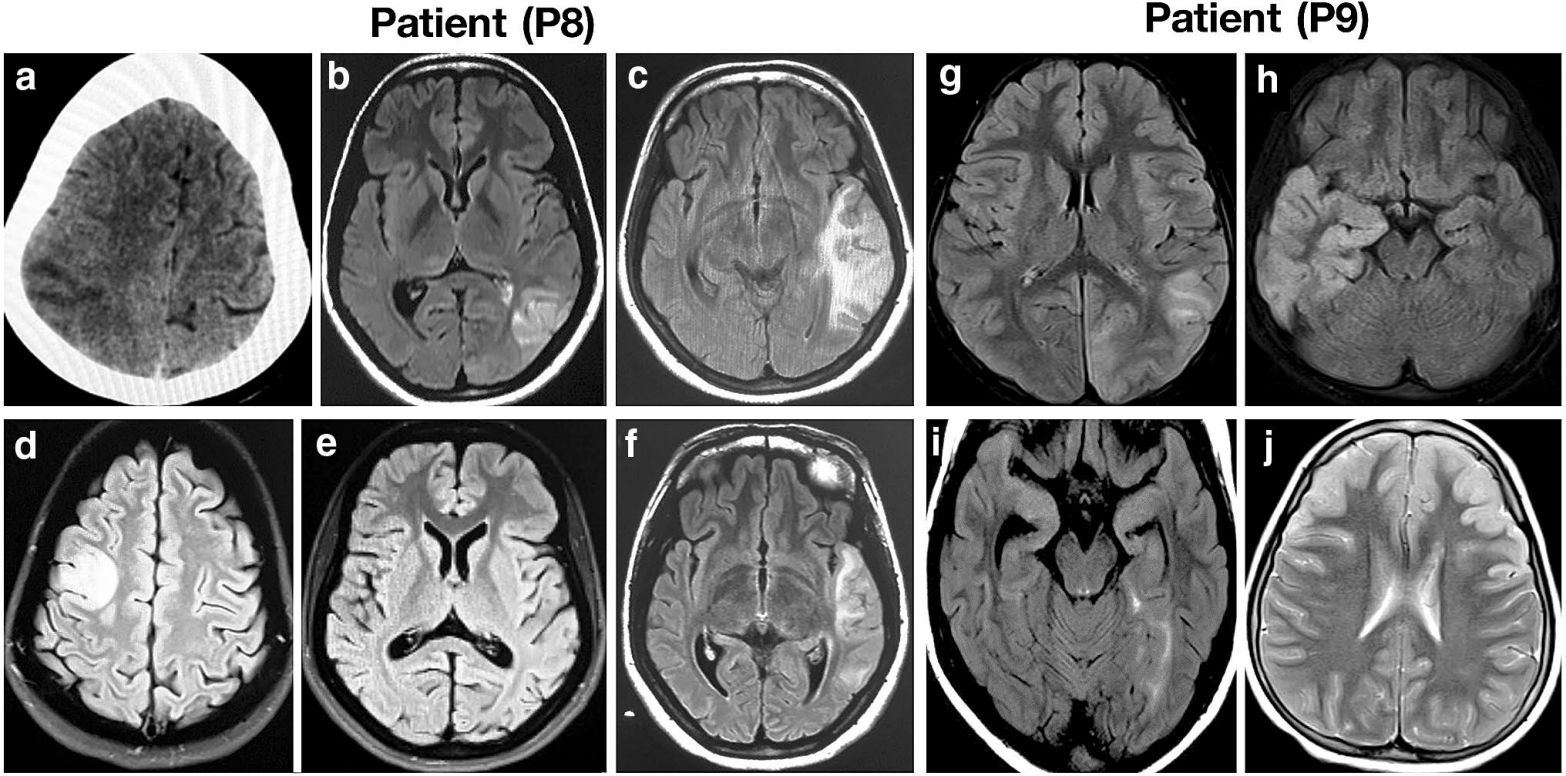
CT and MRI brain of MELAS patients with nuclear gene mutations. Axial sections of CT and MRI of brain show shifting cortical-based signal changes that do not conform any arterial territory in patient P8 (A–F). CT brain shows an ill-defined focal hypodensity in the left parietal region in 2008 (a), Brain MRI shows focal wedge-shaped hyperintensity in the left temporal region resembling an ‘infarct’ in 2010 (b, c), focal hyperintensity in the right frontal region in 2012, while the lesion seen in the left temporal region in 2010 has resolved completely (d, e) and recurrence of focal hyperintensity in the left temporal region in 2014 (f). Axial sections of MRI of brain show shifting hyperintensities involving the cortex that do not conform an arterial territory in patient P9 (g–j). Brain MRI shows focal hyperintensity in the left parieto-occipital region in September 2012 (g), focal hyperintensity in the right temporal region in November 2012 (h), focal hyperintensity in left medial temporal region in January 2013 (i) and hyperintensity in bilateral frontal region in August 2016 (j)
